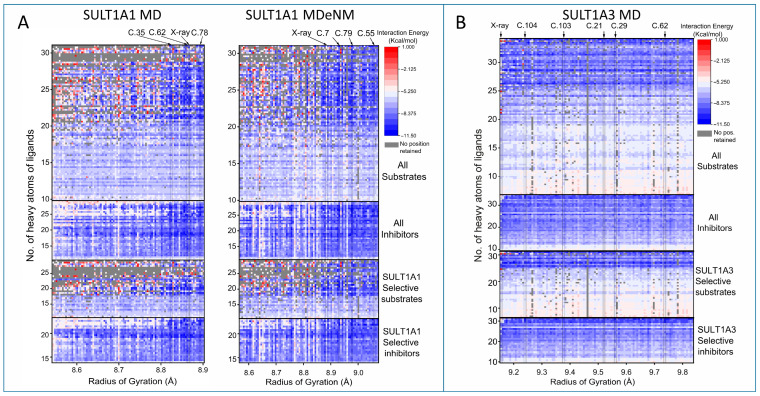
Docking score map of SULT1 substrates and inhibitors into protein cluster centroids. (A) Docking scores of SULT1A1on the MD and MDeNM centroids, ordered by the radius of gyration (RGYR) of the binding pocket (see residues in Supplementary Materials). (B) Docking scores for SULT1A3 on the MD centroids. Ligands in the ordinate axes are ordered by the number of their heavy atoms. Selective ligands indicate ligands not interacting with the other isoform. Blue to white colors indicate good predicted scores (for interaction energy), while red colors represent poor scores. Gray color indicates that docking has not produced a satisfactory pose of the substrate for the catalytic reaction. The results of the top 5 well-performing centroids and the X-ray conformation are marked with arrows.
